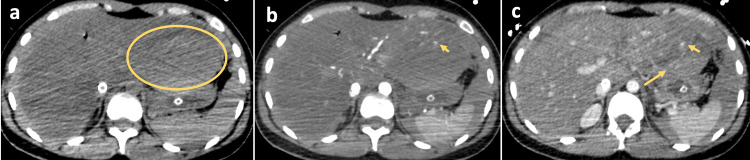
Abdominal computed tomography scan before and after the administration of iodine intravenous contrast medium (a) Non-enhanced axial image showing a spontaneously dense hematoma between the left lobe of the liver and the stomach; enhanced axial images in arterial (b) and portal (c) phases showing active bleeding (arrows) and progressive increase of hematoma density.
